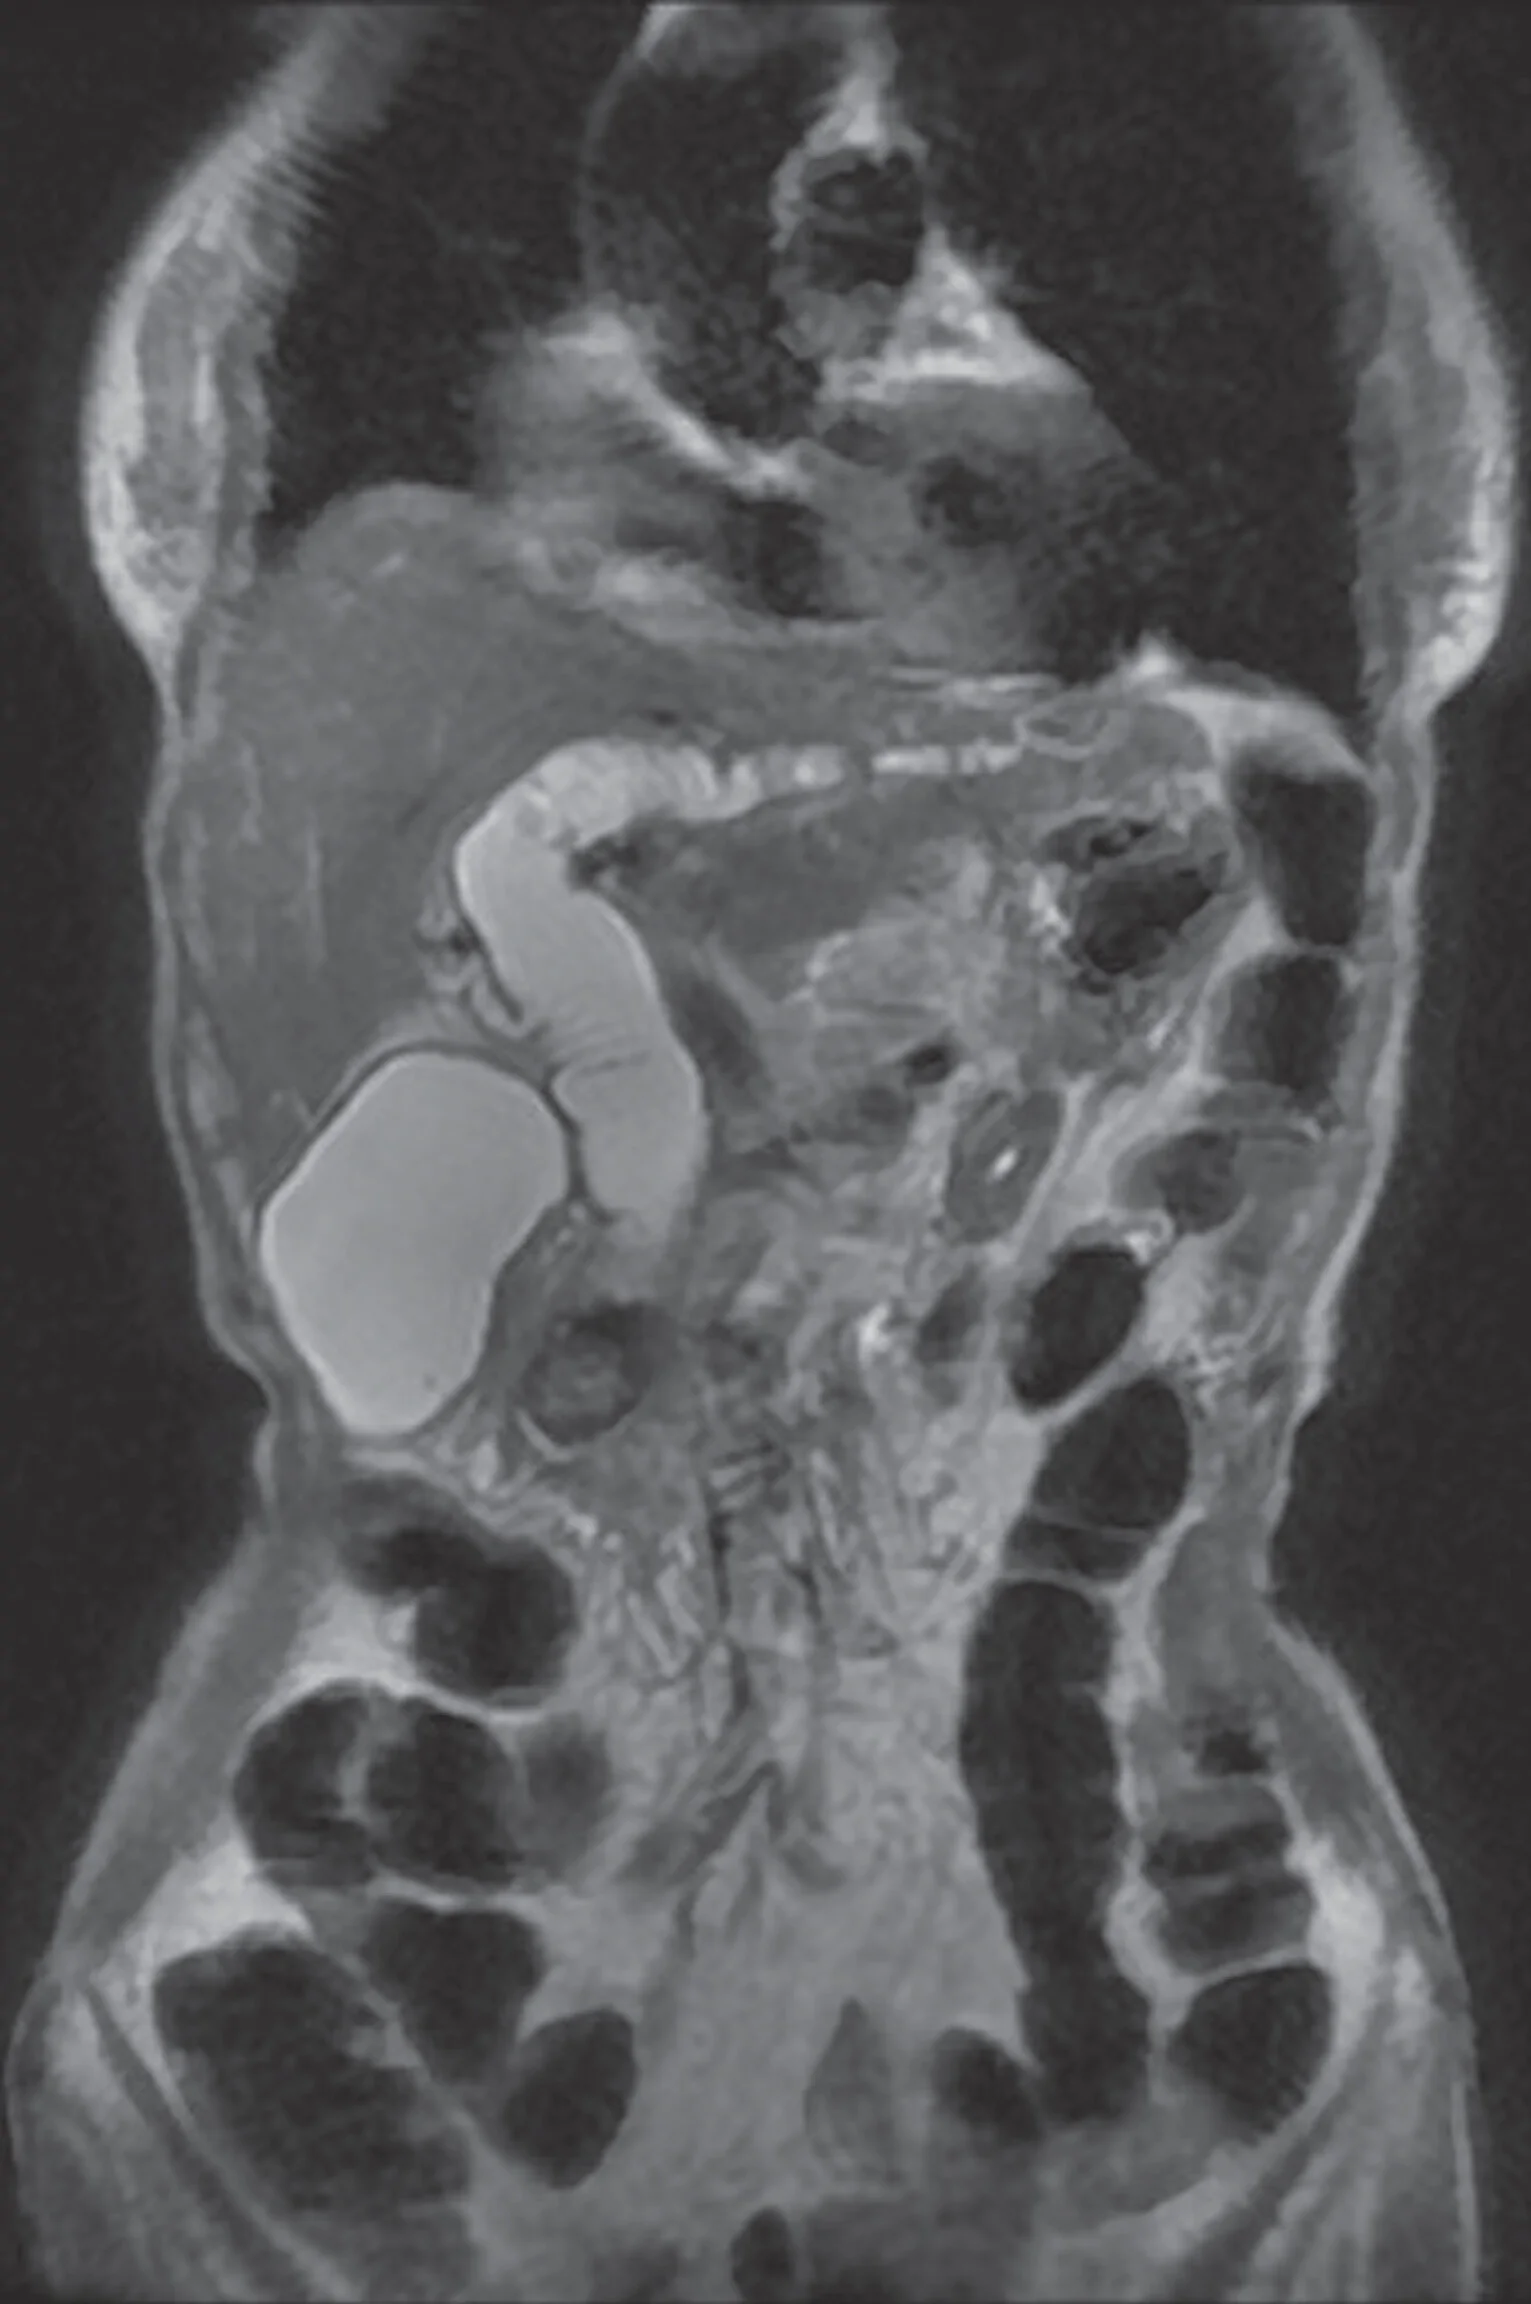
Upper abdominal magnetic resonance image showing a 2×3-cm stone
